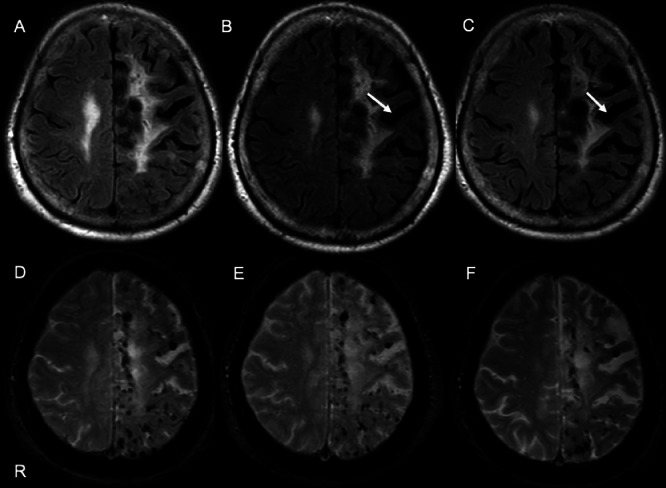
Fluid-attenuated inversion recovery (FLAIR) images obtained at initial presentation (A), 1-year follow-up (B), and 3-year follow-up (C) demonstrate gradual enlargement of the left cerebral sulci (white arrow), indicating progressive left hemispheric atrophy over time. T2*-weighted images obtained at the same time points (D–F) show multiple cerebral microbleeds predominantly distributed in the left hemisphere, with no increase in number or change in spatial distribution during follow-up. These findings indicate that structural atrophy progressed independently of microbleed burden. The letter ‘R’ indicates the right side of the patient in all images.
